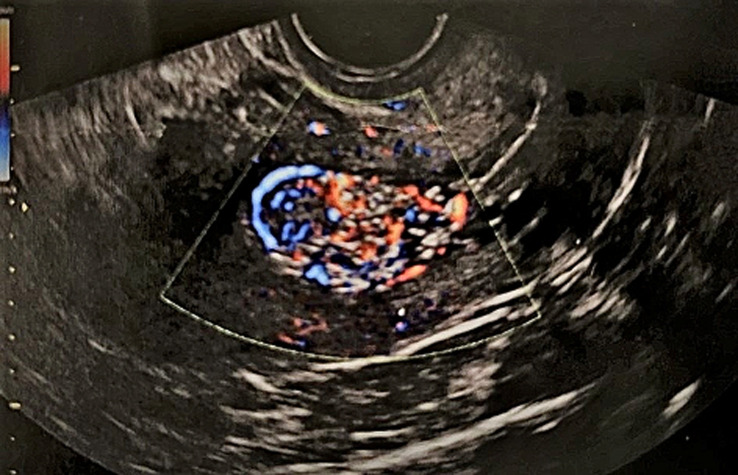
transvaginal Doppler ultrasound imaging of an atypical polypoid adenomyoma of the uterus; the imaging of increased vascularity of the endometrial mass
